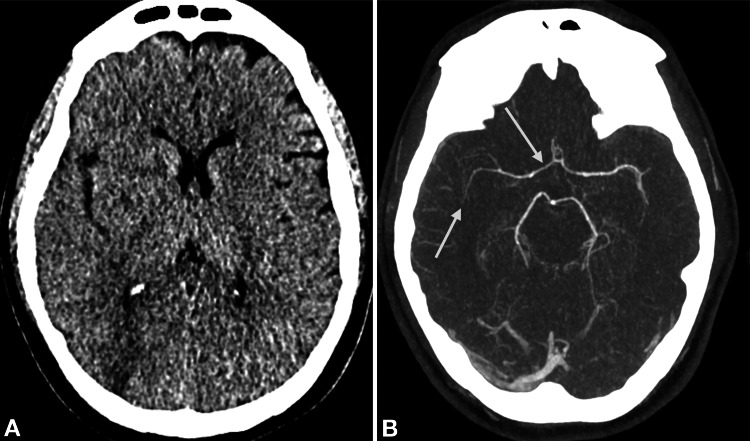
Initial native computed tomography and computed tomography angiography of the brain (A) Native computed tomography shows demarcated ischaemia in the right posterior and inner border zone, as well as frontoparietally on the right-hand side, along with an oedematous right cerebral hemisphere. (B) Computed tomography angiography reveals severe, multifocal vessel narrowing (arrows), most pronounced in the right middle cerebral artery.
